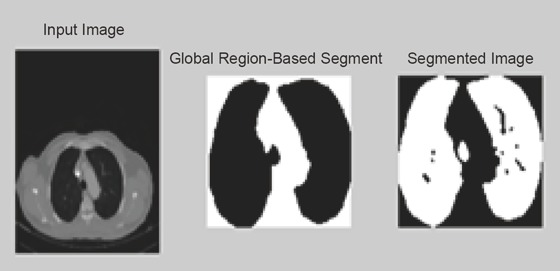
Segmented 2-D CT image using Region Growing.
